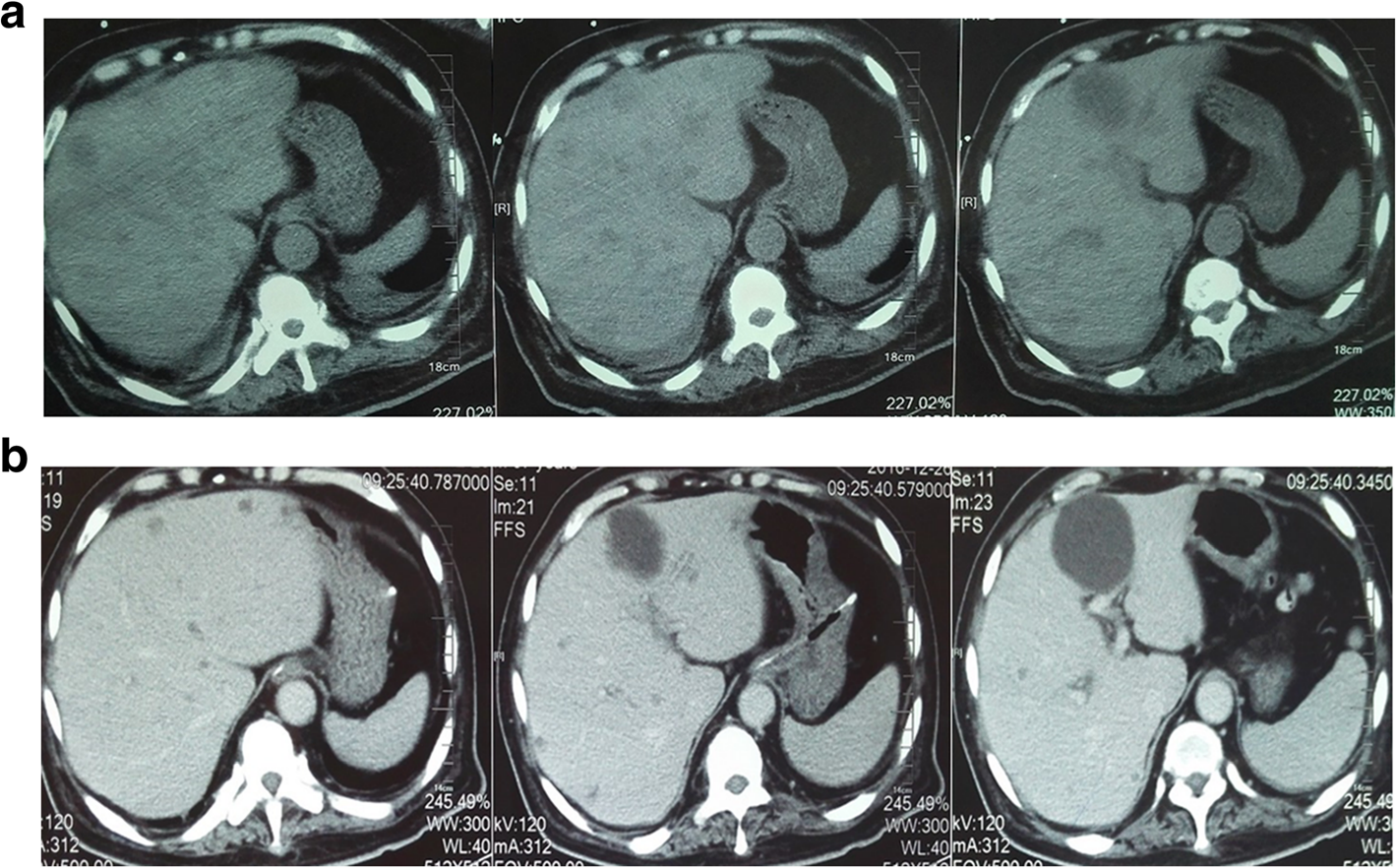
Shrinkage of the patient’s intra-hepatic lesions after two months of olaparib treatment. CT scan of the abdomen before (a) and after (b) two months of olaparib treatment
